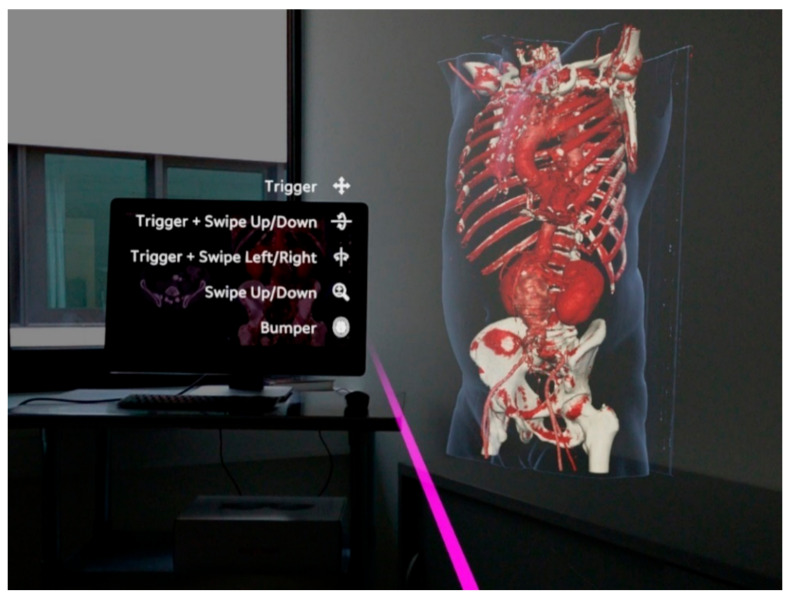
Observer’s point of view using the Magic Leap 1 (Plantation, Magic Leap, FL, USA) and the Elements Viewer software (Brainlab AG, Munich, Germany). The 3D virtual model on the right is based on a computed tomography angiography (CTA) of a patient with an abdominal aortic aneurysm. The virtual pink pointer is generated in front of the manual controller and can be used to interact with the virtual model and the user interface of the software.
